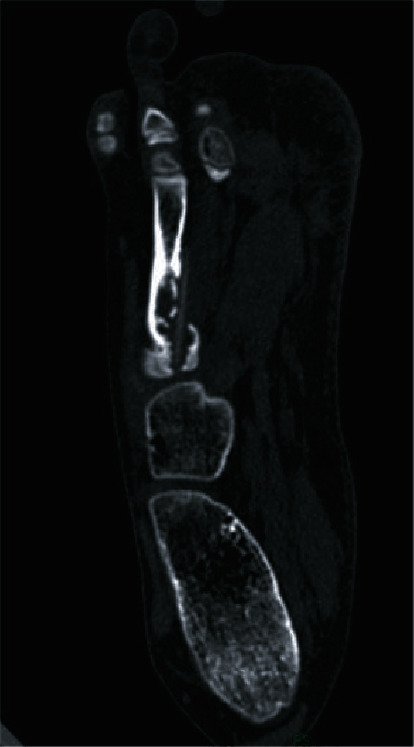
Transversal computed tomography key image with a linear hyperintense configuration, suggesting the presence of a foreign body.
